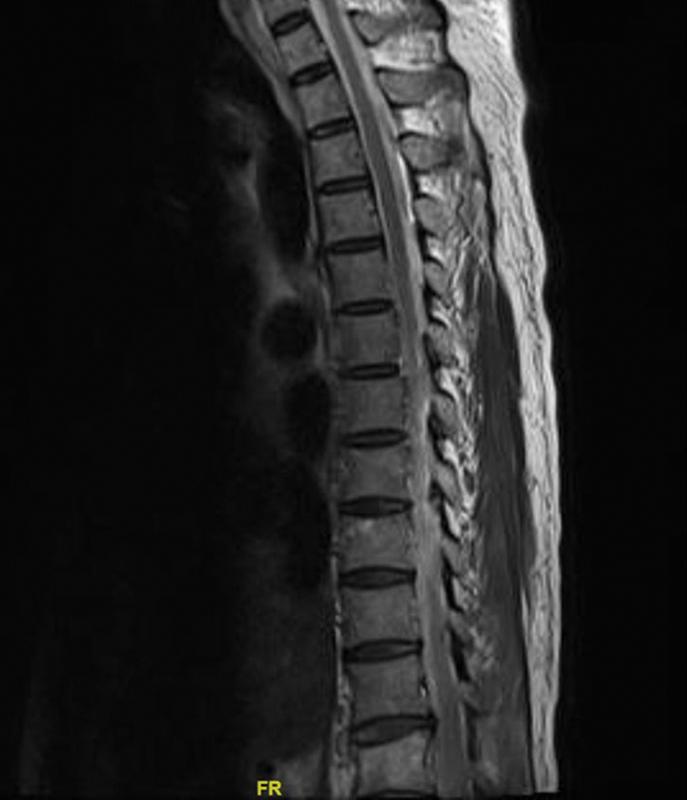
Postoperative magnetic resonance imaging spine which was normal.
